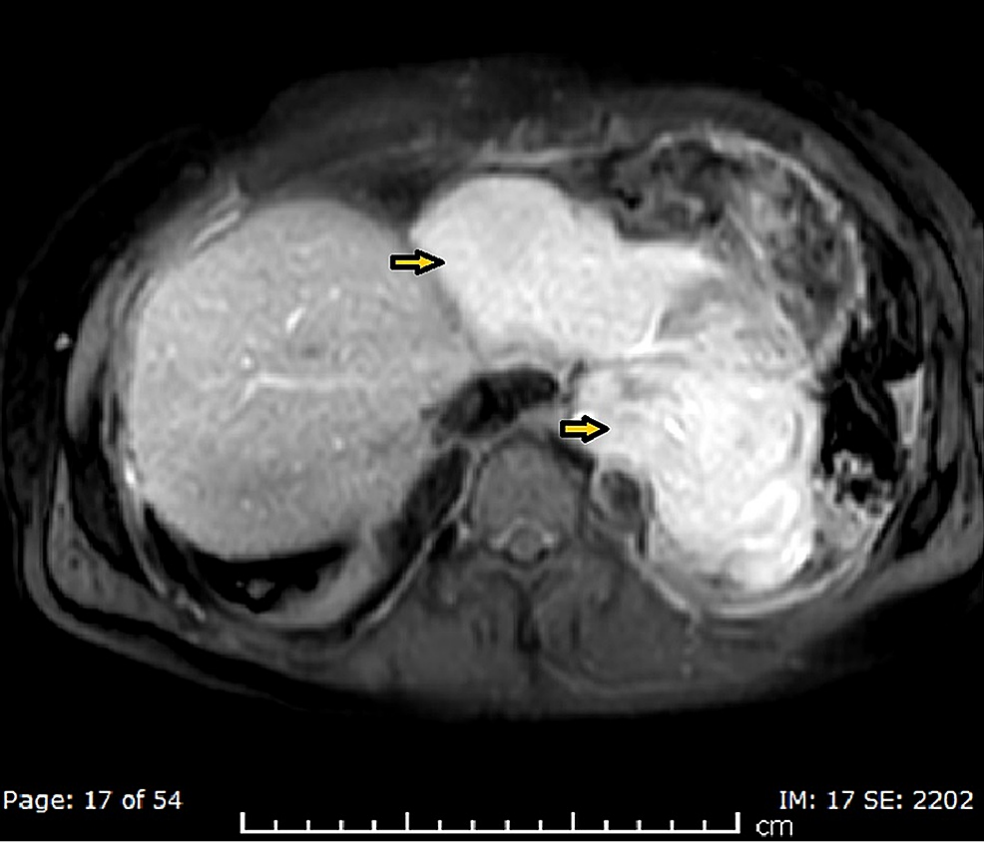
MRI post-contrast axial image showing a pericardial mass lesion (arrowheads)
